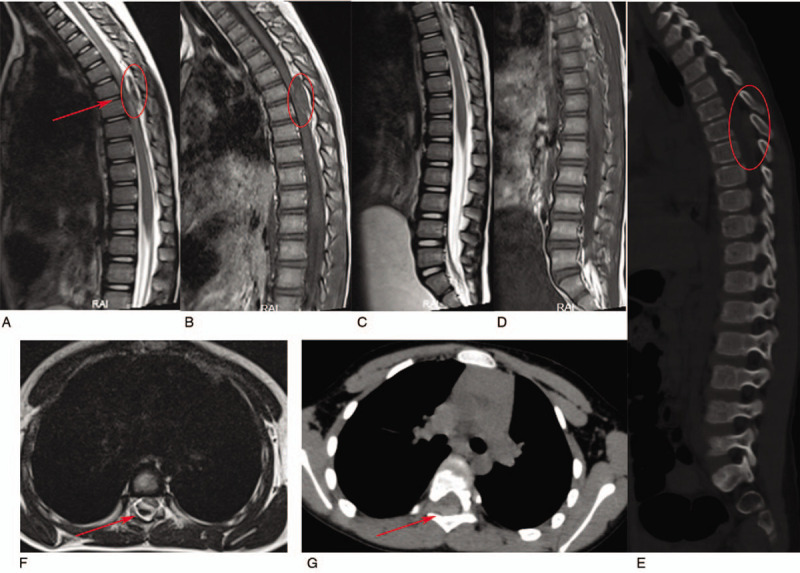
Preoperative images. The magnetic resonance imaging and computed tomography showed an abnormal epidural mass (28 × 9 mm) at the level of T6-7 (red circle and red arrow).
